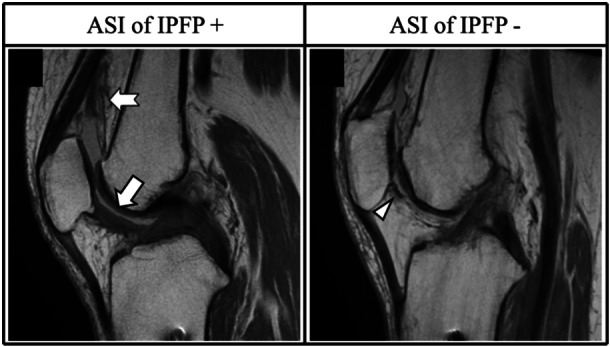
Magnetic resonance imaging findings of abnormal signal intensity (ASI) of the infrapatellar fat pad. (a, b) Sagittal images of the knee flexed at 10° at 1 year postoperatively. (a) Continuous increased low signal intensity between the patella and anterior cruciate ligament insertion (arrow). Mostly concomitant ASI of the suprapatellar pouch was found (swallow‐tail arrow). (b) Focal area of low signal intensity (arrowhead).
